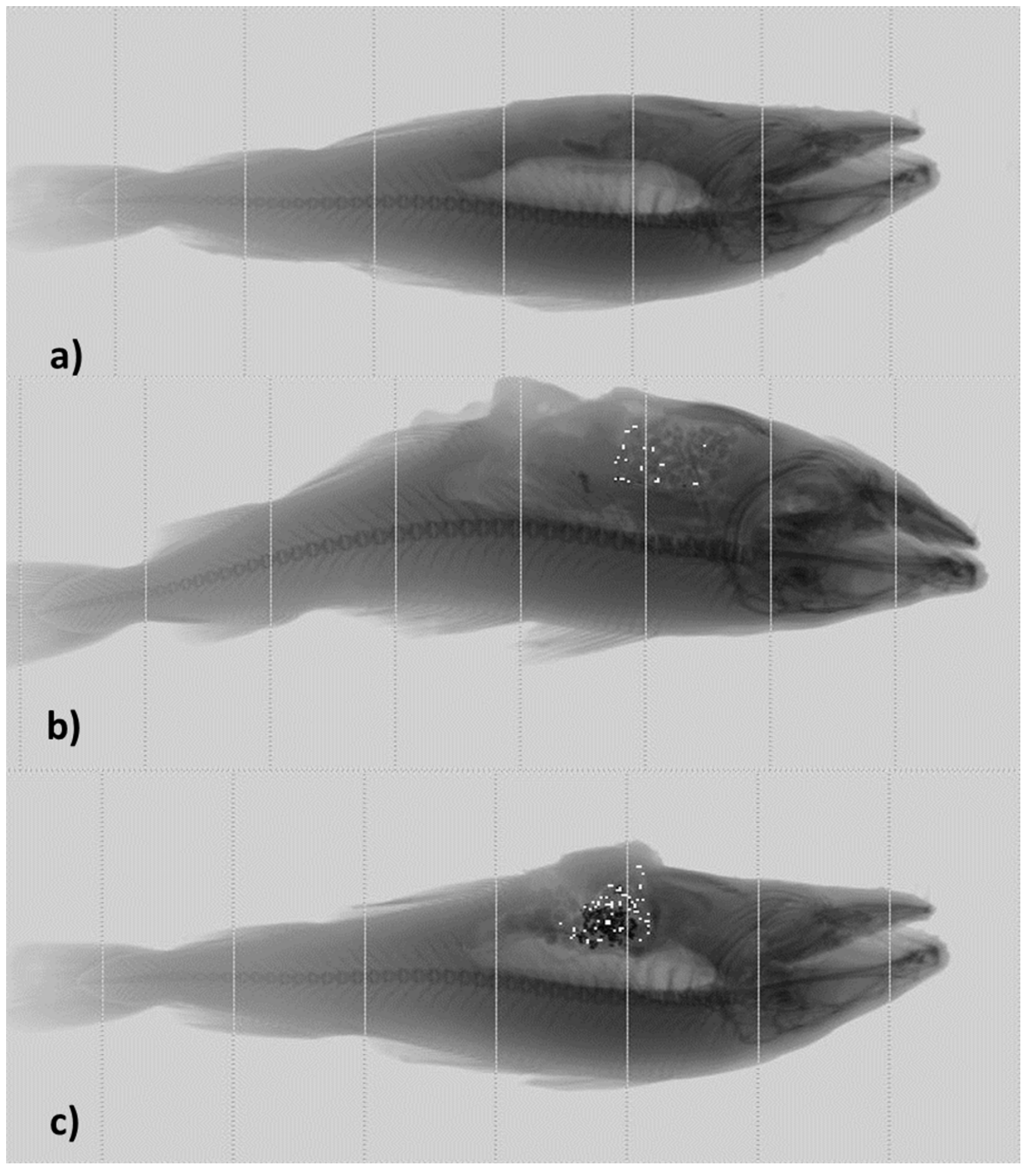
X-ray images of Atlantic cod generated from the Ishida X-ray machine: a) image of fish without feed, b) image of fish with Chrome-Nickel (CrNi) markers at 0.1% (0.03 g) weight percent of total feed content in gut; c) image of fish with Chrome-Nickel (CrNi) markers at 10% (3 g) weight percent of total feed content in gut.
